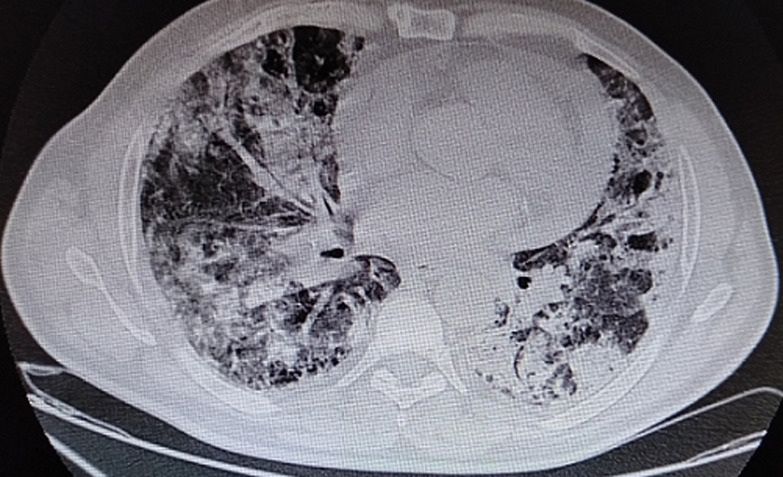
chest CT scan in axial window showed signs of COVID-19 pneumonia
